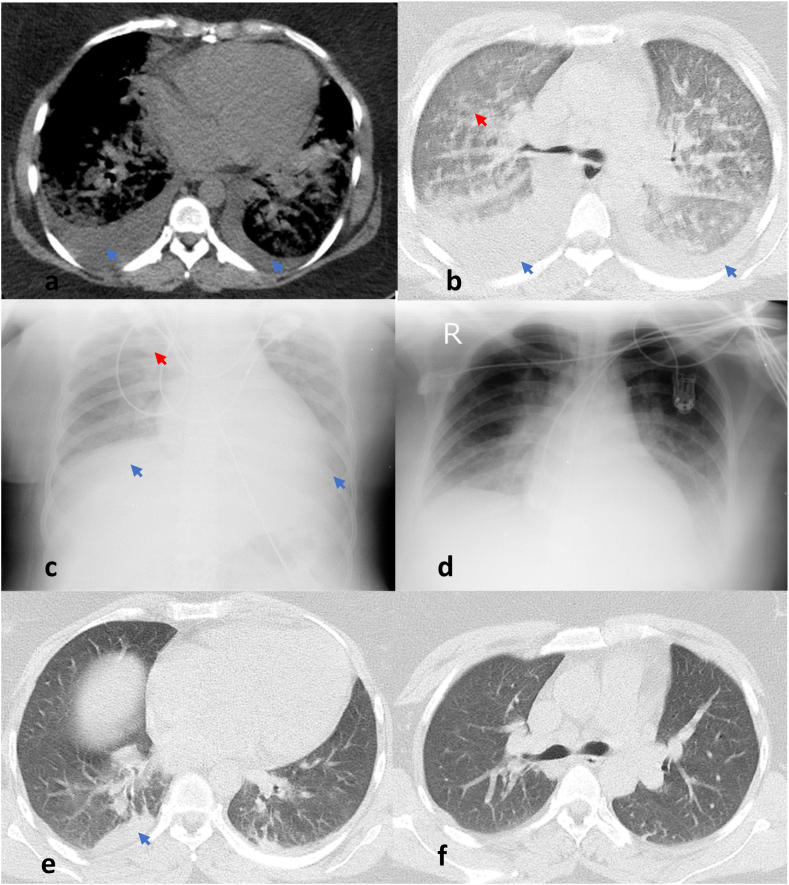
Patient's chest Imaging. a, b, chest CT scan at the first day of admission after laryngospasm displaying bilateral pleural effusion (Blue arrow) and bilateral ground glass opacities (Red arrow). c, d, chest radiography at the second and third day of admission. (For interpretation of the references to colour in this figure legend, the reader is referred to the Web version of this article.)
